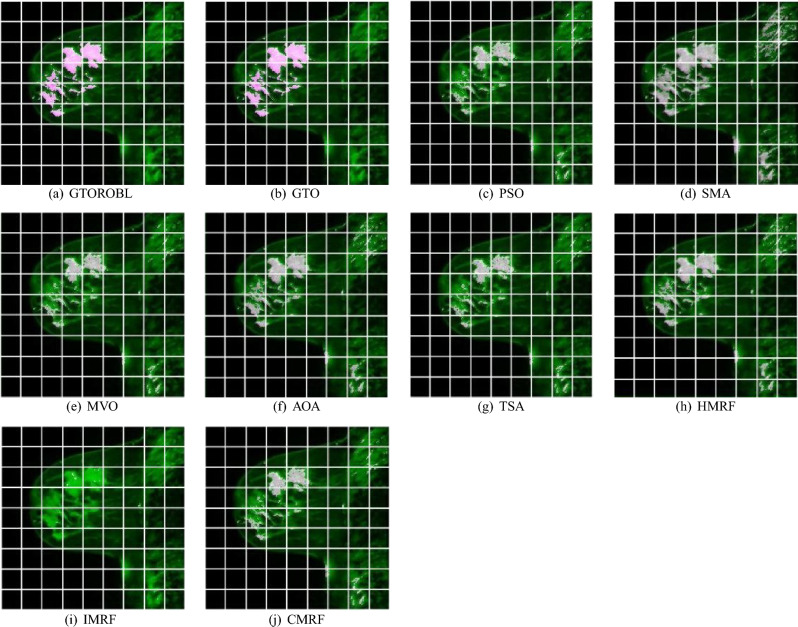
Localized lesions (bright colored spot) in MR images for patient-2 (a) GTORBL, (b) GTO, (c) PSO, (d) SMA, (e) MVO, (f) AOA, (g) TSA, (h) HMRF, (i) IMRF, and (j) CMRF.
